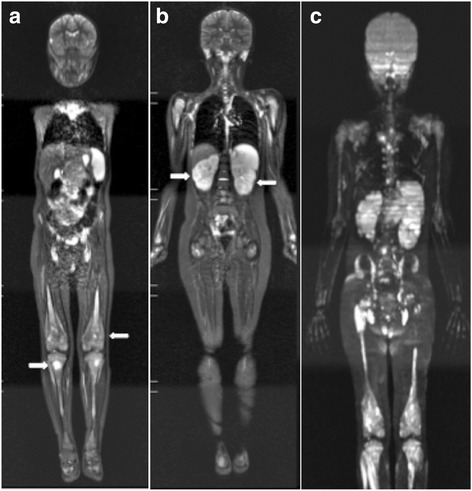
Whole-body MRI was performed in a 6-year-old male patient with acute lymphoblastic leukemia for staging. a Diaphyseal and metaphyseal lesions in the distal femoral and proximal tibial regions are represented by hyperintense signals in a coronal STIR sequence (*arrows*). b Signs of bilateral renal infiltration by the underlying pathology (*arrows*) were observed. c A diffusion sequence demonstrated multiple hyperintense foci, consistent with leukemic infiltration
